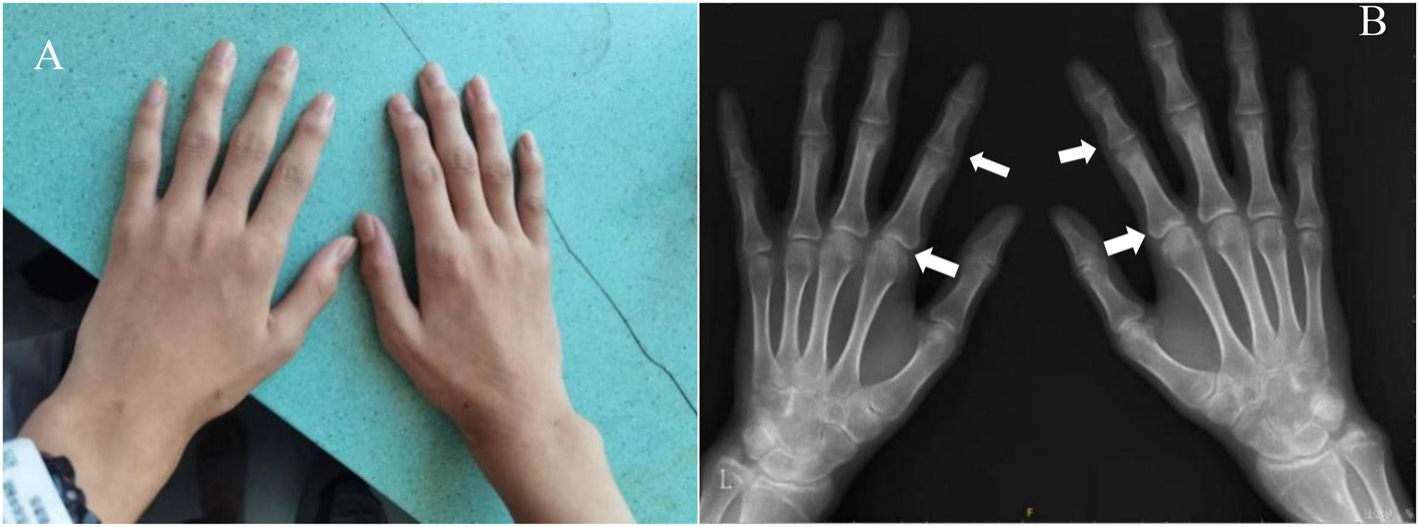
(A) Spindle-shaped enlargement of proximal and distal finger joints of both hands; (B) hands x-ray showing enlarged epiphyses and metaphyses of the metacarpals and phalanges, with joint spaces narrowing and osteophytic formations (arrows). The bone age of the patient was 16 years old. No significant inflammatory changes were observed.
